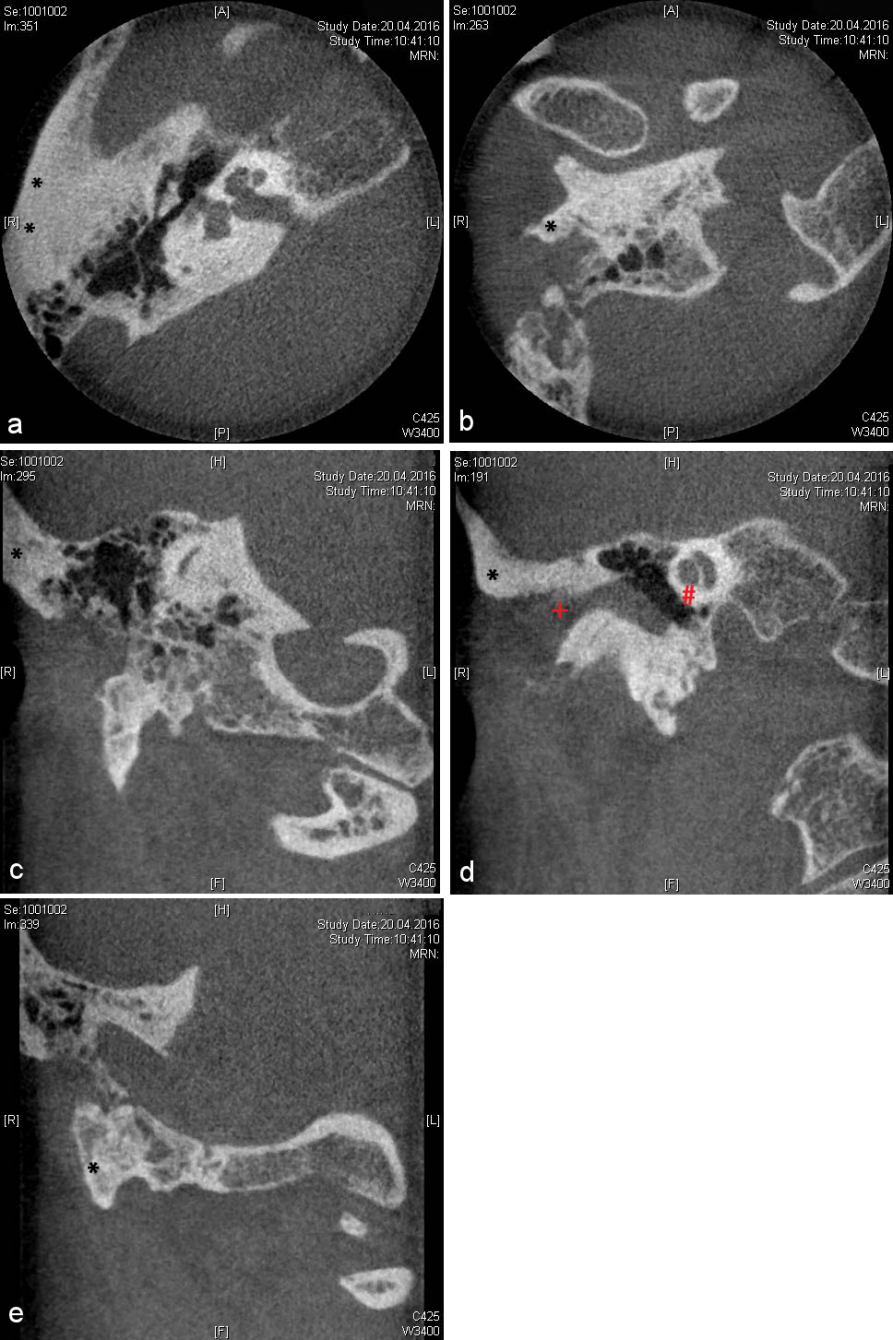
Preoperative cone beam computerized tomography (CBCT) imaging. a: Axial section. Black asterisk indicating planned cranial implant positions. b: Axial section. Black asterisk indicating planned caudal implant position. c: Coronal section. Black asterisk indicating planned first cranial implant position. d: Coronal section. Black asterisk indicating planned second cranial implant position, red plus marking the external meatus acusticus, and red diamond showing the cochlea. e: Coronal section. Black asterisk indicating planned caudal implant position.
